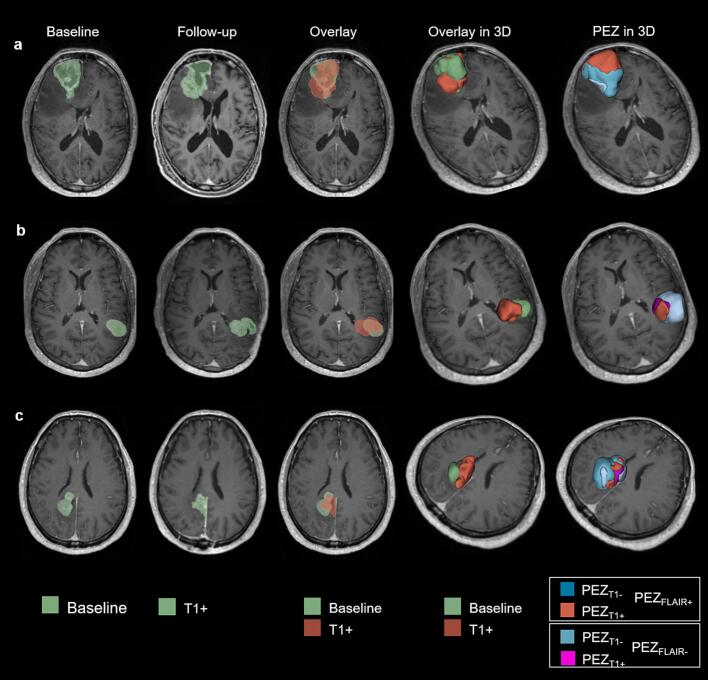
Examples of follow-up MRI registration to baseline scans and corresponding 3D segmentations of the *peri*-enhancing zone (PEZ) in three patients with glioblastoma. (a) 69-year-old male with recurrence 6.5 months after diagnosis; (b) 69-year-old female with recurrence 4.5 months after diagnosis; (c) 55-year-old male with recurrence 7.5 months after diagnosis. All patients underwent a second resection following follow-up MRI.
